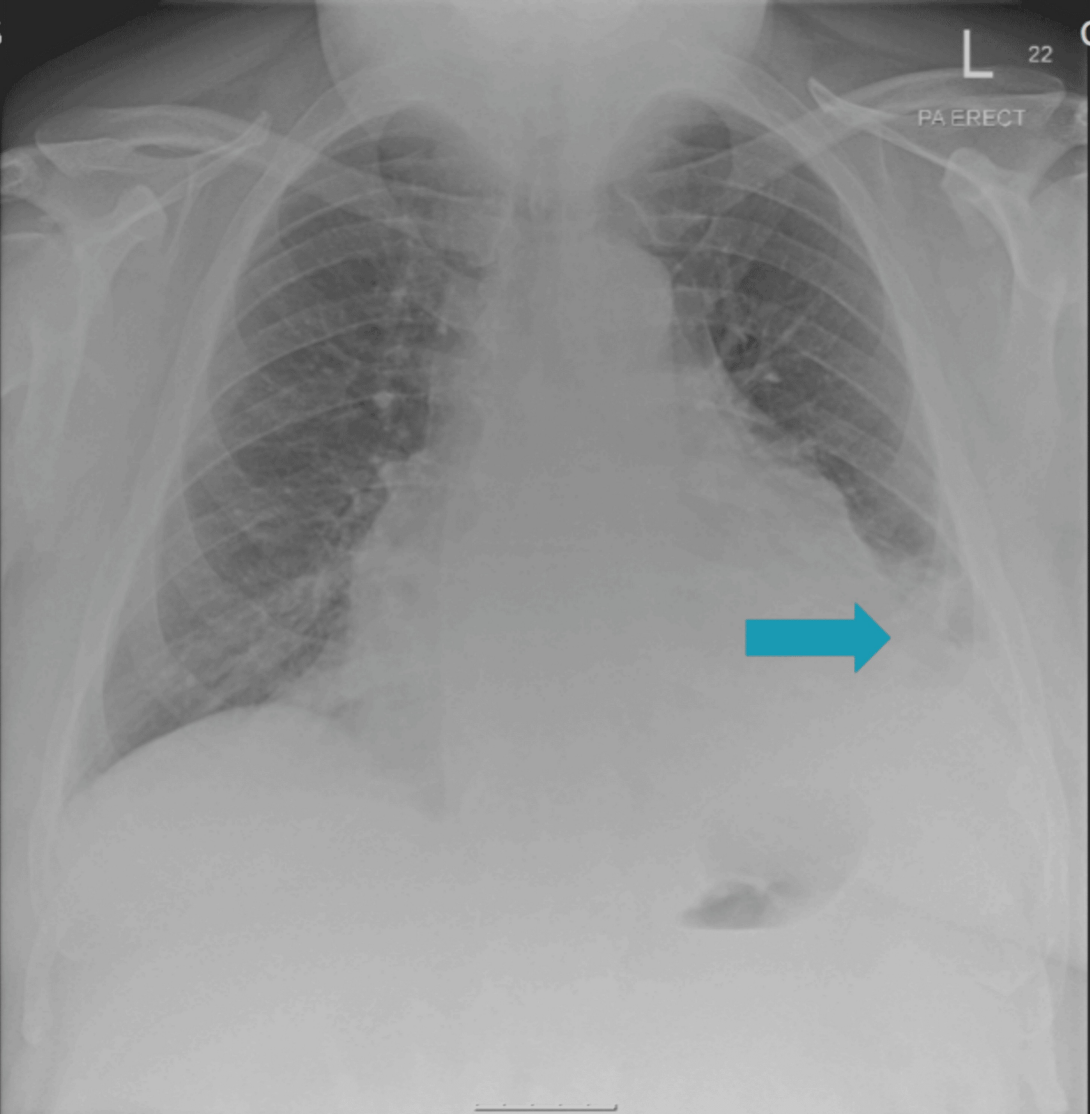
Postero-anterior chest radiograph demonstrating an enlarged cardiac silhouette with blunting of the left costophrenic angle, suggestive of a moderate pericardial effusion.
